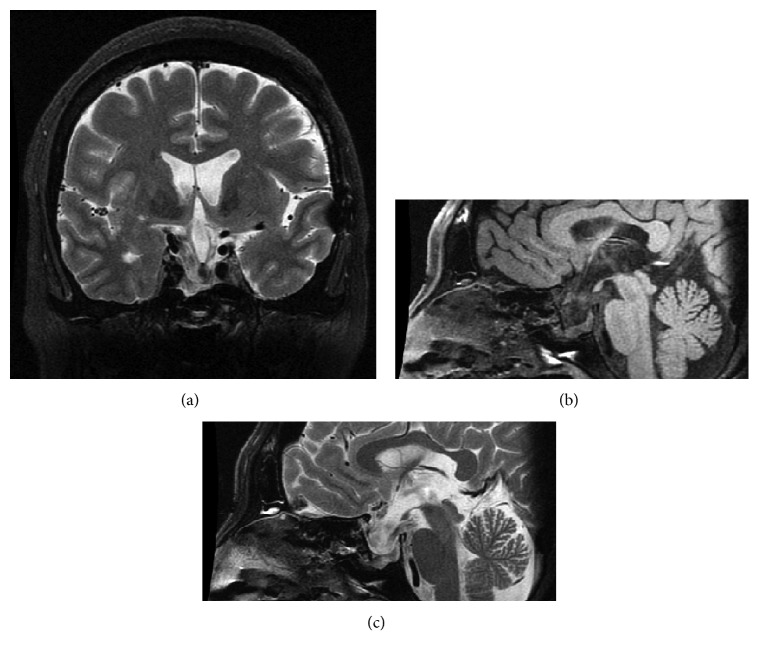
Follow-up MRI of T2-coronal (a), T1-sagittal (b), and T2-sagittal (c) at 10 years after the initial operation showing that the suprasellar cyst and sellar tumor were still completely controlled.
